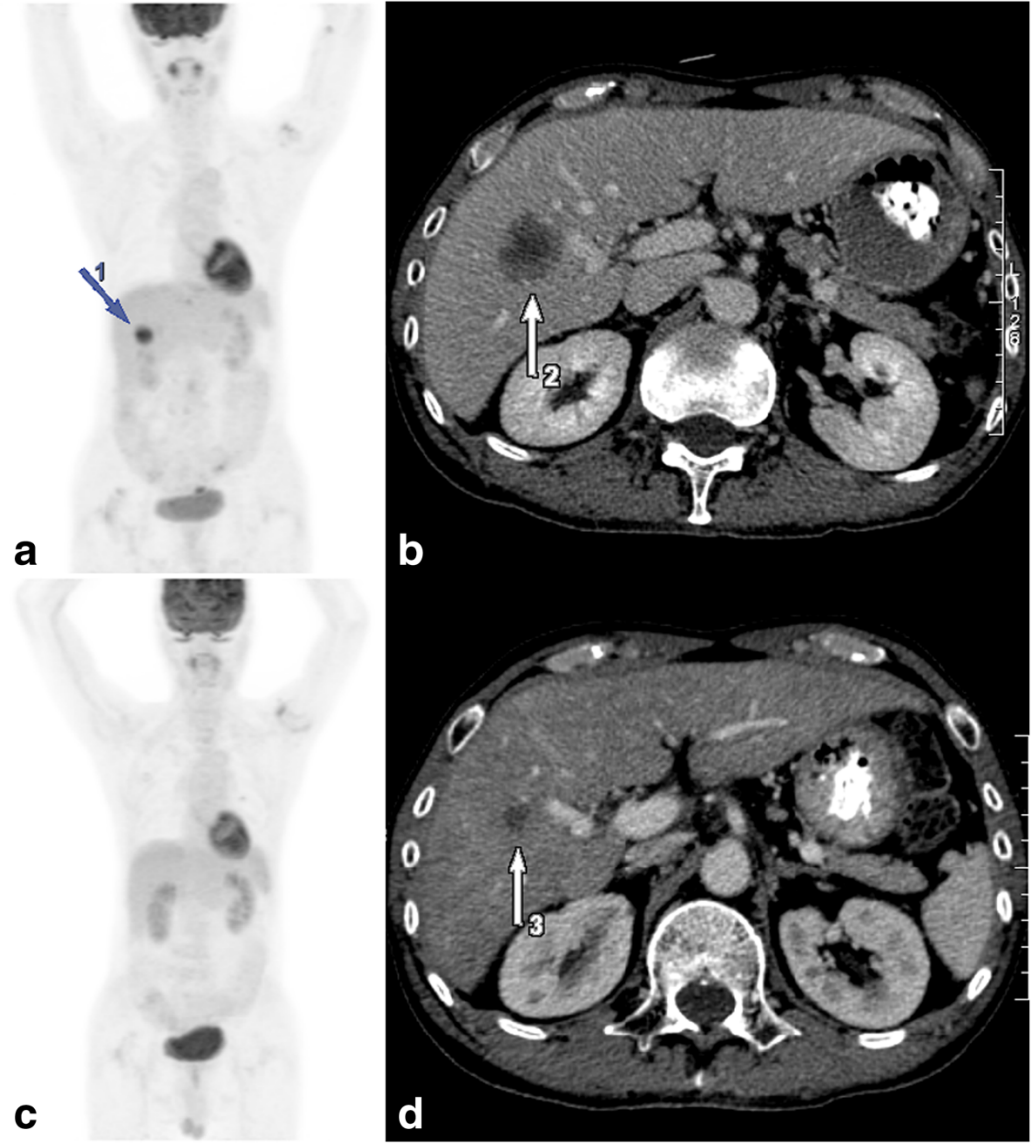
Discordance between PERCIST and RECIST criteria in a 66-year-old male patient with kras wild-type T4N1M1 recto-sigmoid adenocarcinoma. Top row: PET and CT images performed at baseline after surgical resection of the primary tumor. a Maximum-intensity-projection (MIP) image shows a hypermetabolic mass in segment V/VIII of the liver (blue arrow 1). b Corresponding contrast enhanced CT image confirming the presence of a liver metastasis (white arrow 2). Bottom row: PET and CT images after 10 cycles of XELOX and cetuximab. c MIP image shows CMR compared to (d) CT image demonstrates a residual lesion (white arrow 3). The patient was considered to have partial response to treatment by RECIST criteria. The patient was considered to have progressive metabolic disease after 5 months of complete metabolic response
